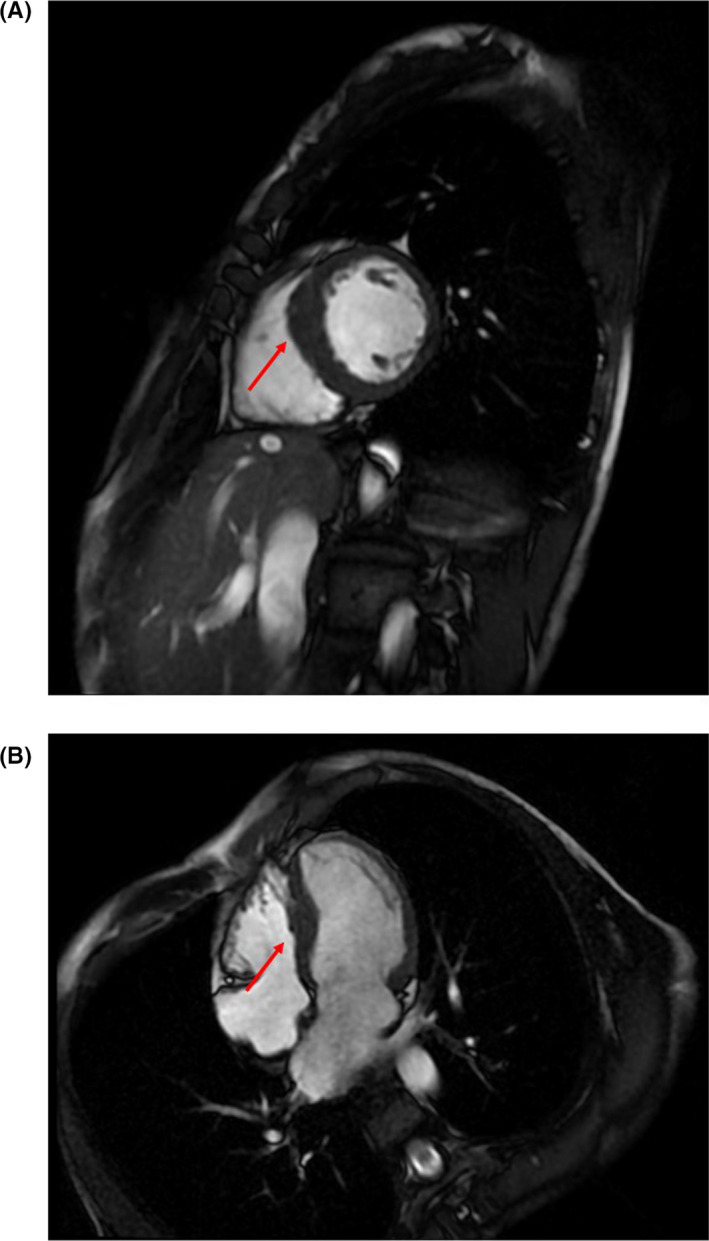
Cine magnetic resonance imaging of the patient with swallowing‐induced atrial tachycardia and hypertrophic cardiomyopathy. A, The short‐axis view shows localized mild hypertrophy of the interventricular septum (red arrow). B, The four‐chamber view also shows localized mild hypertrophy of the interventricular septum (red arrow)
